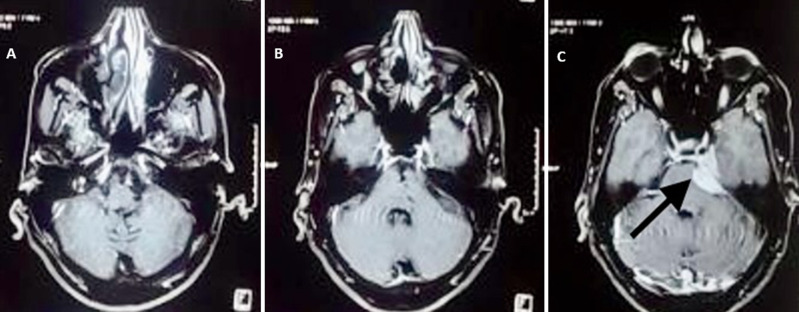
A, B, C) magnetic resonance imaging of the brain, causing post-contrast enhancement with a positive dural trail sign
